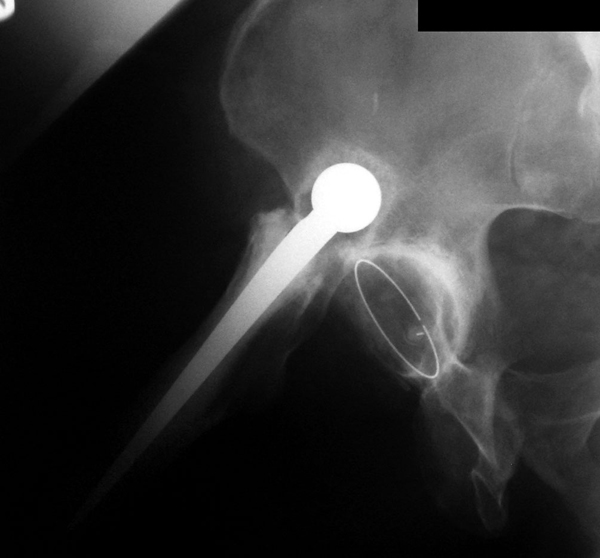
A radiograph of the right lateral hip showing the dislocated right Exeter total hip replacement, with the prosthetic femoral head articulating freely within a neoacetabulum.
